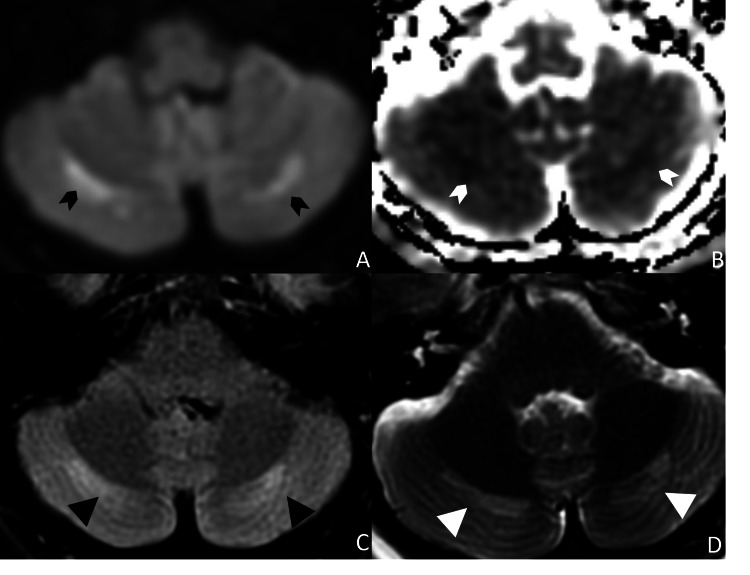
Opioid-associated amnestic syndrome (OAS) - cytotoxic edema in the cerebelli Axial DWI (A) and ADC map (B) demonstrated symmetric mild restricted diffusion of bilateral cerebelli with associated FLAIR and T2 hyperintensities on the axial FLAIR (C) and T2 (D) sequences. DWI: Diffusion-weighted imaging; FLAIR: Fluid-attenuated inversion recovery
